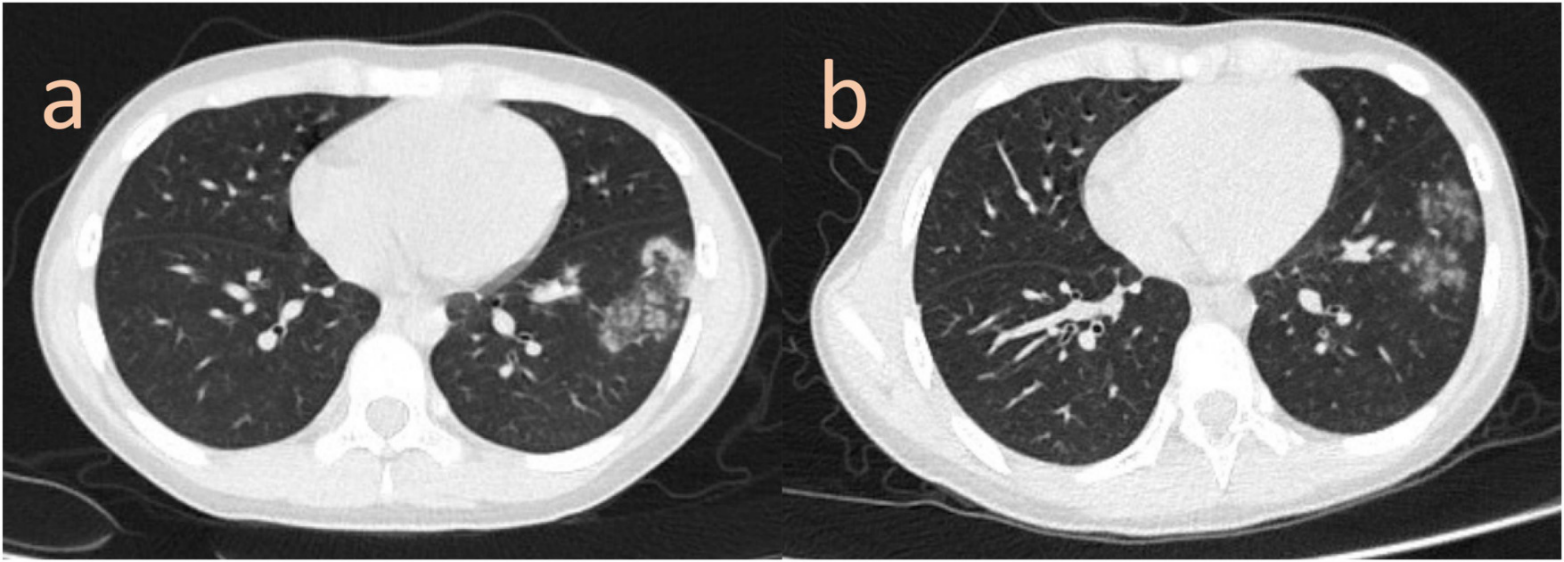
(a) the CT thorax demonstrated new nodules in a different location of the left lower lobe. (b) The lung injury resolved spontaneously after one month.
